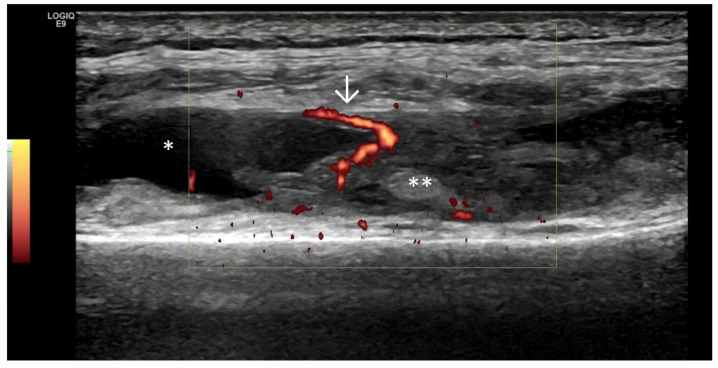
Ultrasonographic suprapatellar longitudinal scan of the knee joint. Against the background of the intra-articular hydrops (asterisk), which is presented as an anechoic zone, the hypoechoic synovial proliferation (two asterisks) with a positive Doppler signal (arrow), an indicator of inflammatory activity, is visible.
